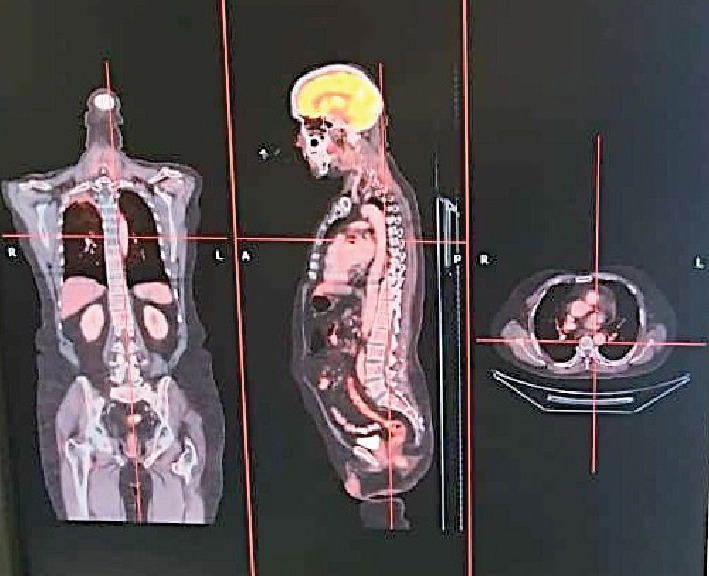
PET-CT showing complete response after 10 cycles of atezolizumab, (sustained for the past 6 years).
